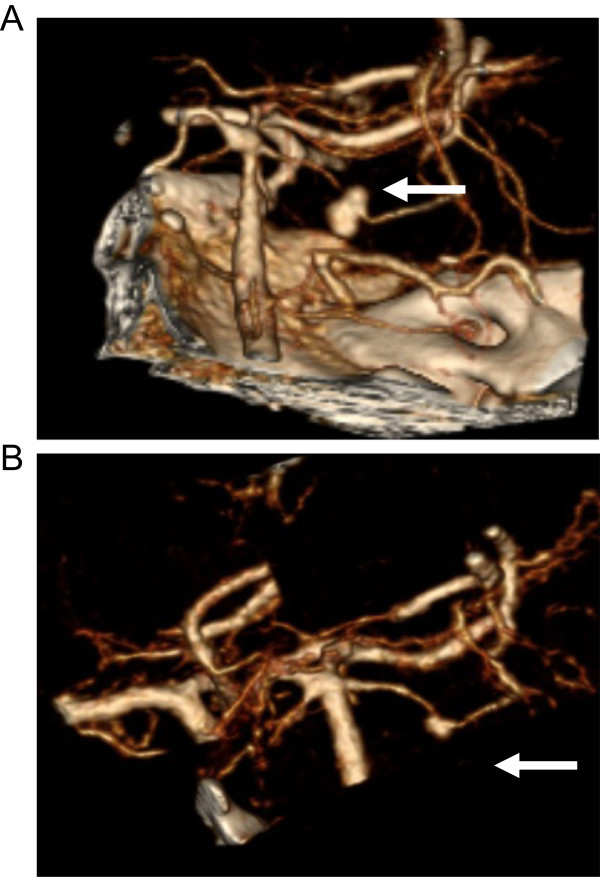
Superior cerebellar artery aneurysm. Reformatted 3-D CT angiography of right superior cerebellar artery showing A. a 4.2 mm aneurysm (arrow) on Nov 5, 2012 and later B. with significant resolution of aneurysm (arrow) after 3 months of amphotericin therapy on Feb 5, 2013.
